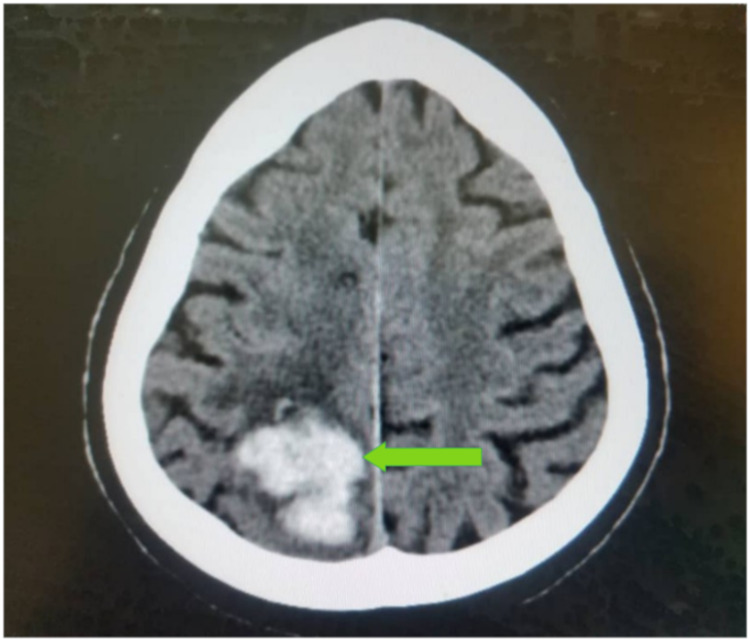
An axial non-enhanced CT scan of the brain of a 42-year-old man with a history of uncontrolled hypertension, who presented with a sudden onset of left-sided hemiplegia and blurred vision, demonstrating a hyperdense lesion in the right occipital lobe with acute blood attenuation with minimal perilesional edema (green arrow) in keeping with right occipital acute hemorrhage.
